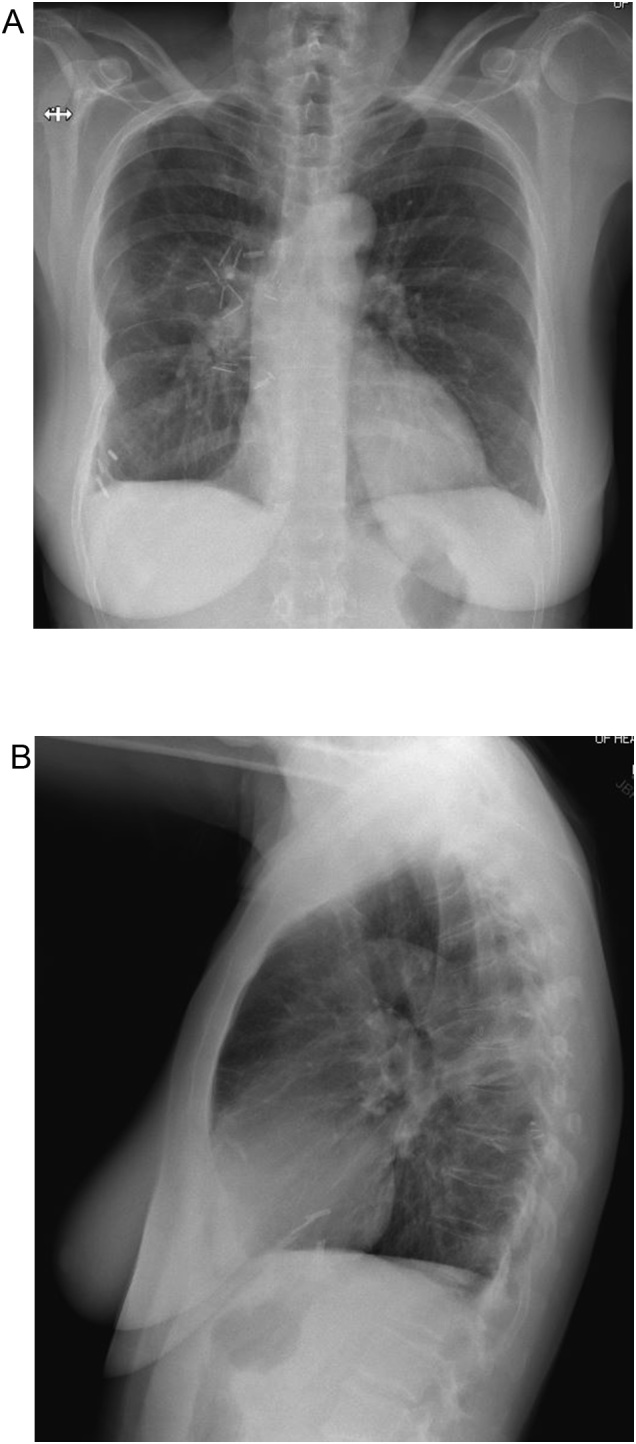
Post-operative chest radiograph (1A: Anterior-posterior view, 1B: Lateral view).
